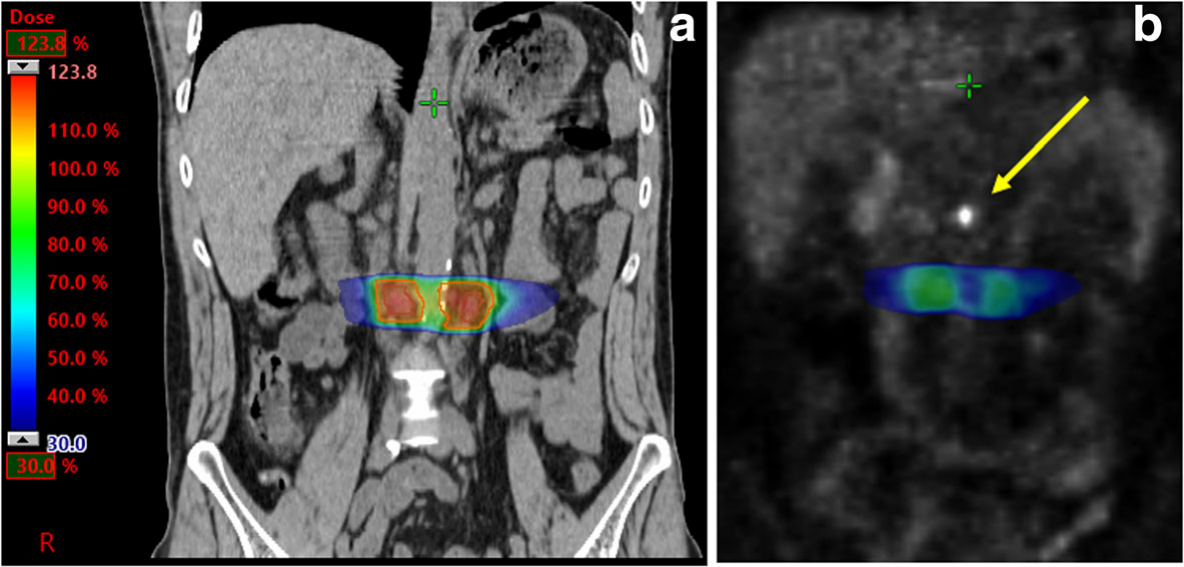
a Coronal image of the dose distribution of radiotherapy. The patient received 3 × 6 Gy @ 80%. b Image fusion of FDG-PET and treatment plan showing 30% of the prescribed dose (blue) in relation to the untreated FDG-positive lymph node (indicated by the yellow arrow)
